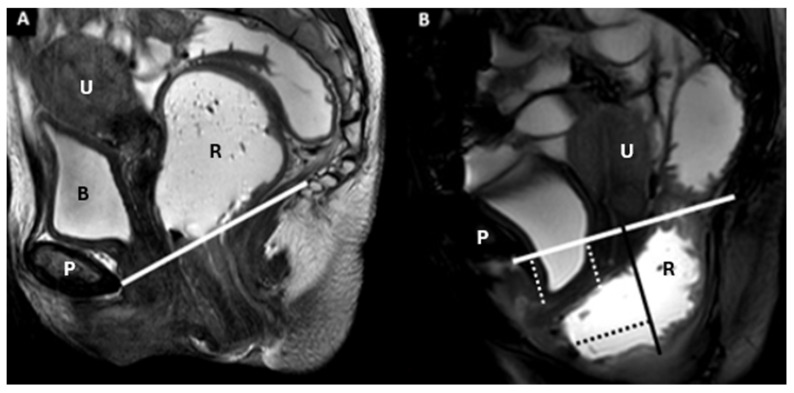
MRI at rest (A) and during defecation (B) showing isthmocele, cystocele, and anterior rectocele. The M line (dark continuous) and PCL (white continuous) are marked. The cystocele and anterior isthmocele are indicated by white dotted lines, while the anterior rectocele is highlighted with black dotted lines. Identifiable structures include the pubic symphysis (P), rectum (R), bladder (B), and uterus (U).
